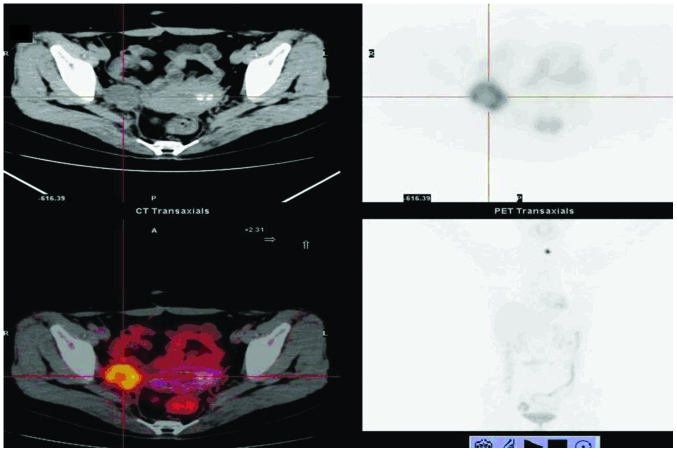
Positron emission tomography/computed tomography (PET/CT) images revealed high-uptake of ^18^F-FDG in the right pelvic lymph node.
